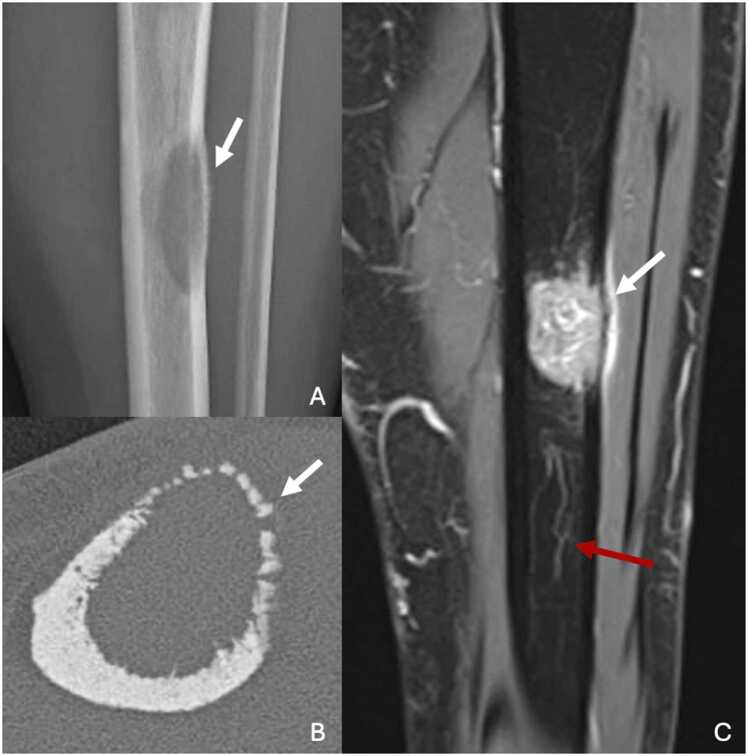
Corticomedullar metastase of the middle third of the left tibia in a 74-year-old woman with a known endometrial neoplasia. A. Face radiography of the left tibia showing a radiolucent oval-shaped corticomedullar lesion with discreet periosteal reaction (white arrow). B. UHR CT image in the axial plane highlighting the periosteal reaction with moth-eaten appearance of the anterior cortex (white arrow). C. T1-weighted fat-suppressed and contrast enhanced MR image in the sagittal plane showing periosteal enhancement adjacent to the metastase, mainly centromedullar. Notice the peritumoral vascularization (red arrow).
